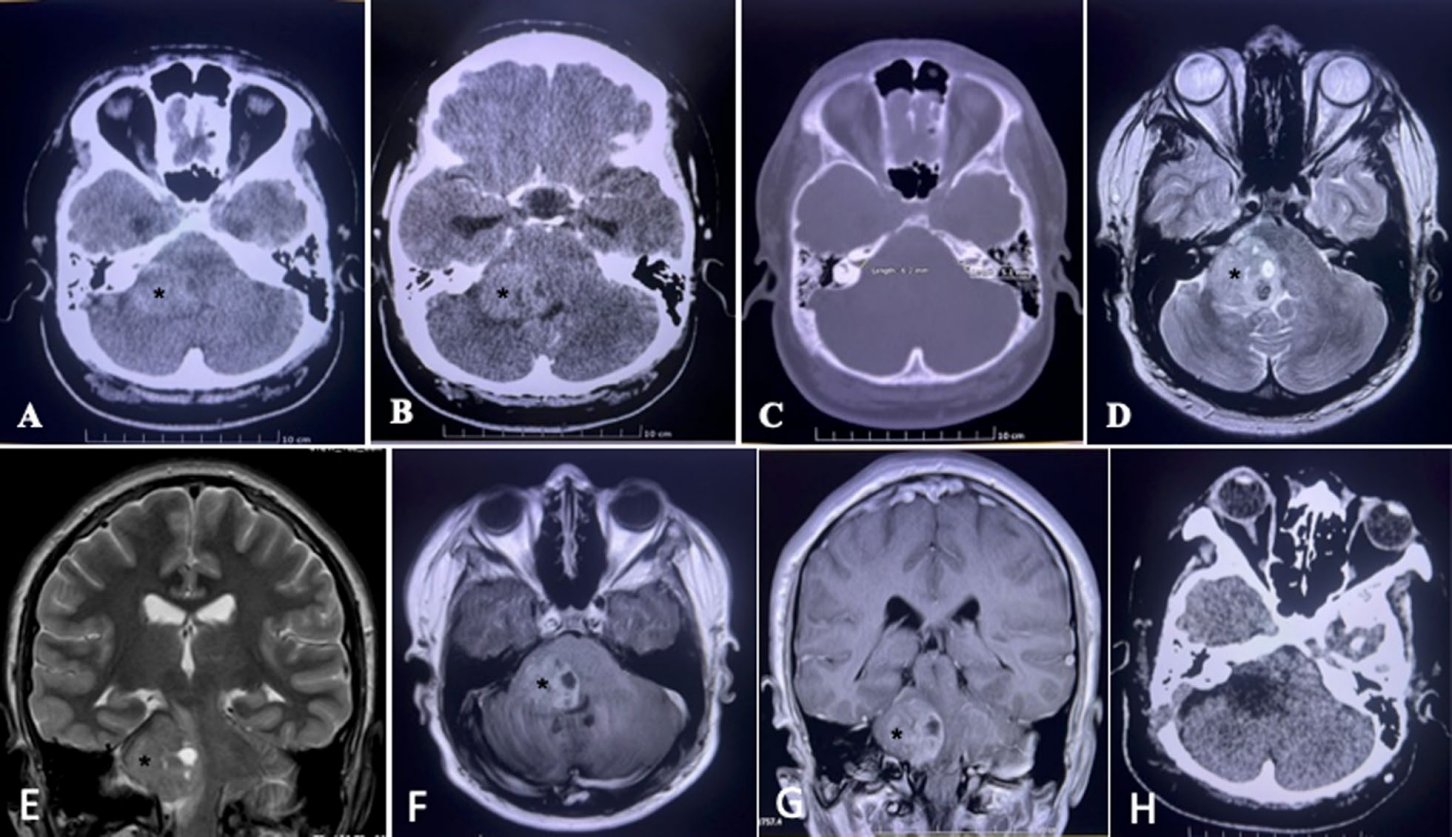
Radiological images. A An axial CT brain revealing a right hypodense CPA tumor (asterisk) that enhances following B contrast sequences, with C IAC enlargement. T2-weighted MR image demonstrating heterogeneous intensity lesions on D axial and E coronal sequence. F, G On the T1-weighted post gadolinium, a heterogenous enhancement is seen following gadolinium. H Day 1 postoperative axial CT brain showing resection cavity with partial relief of brain stem compression
